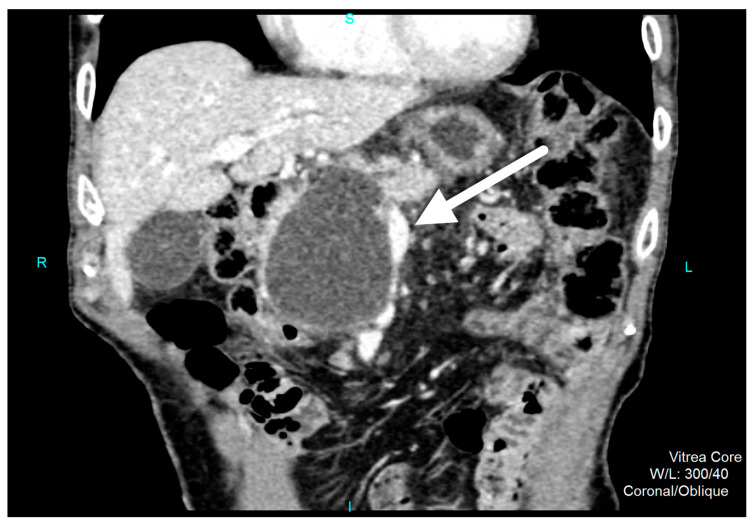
Coronal section contrast-enhanced abdominal computed tomography (CT) shows a well-defined round, encapsulated, necrotic collection that corresponds to walled-off-pancreatic-necrosis (WOPN), with a maximum diameter of 90 mm in the region of the pancreatic head and extrapancreatic extension into the duodeno-pancreatic space.
